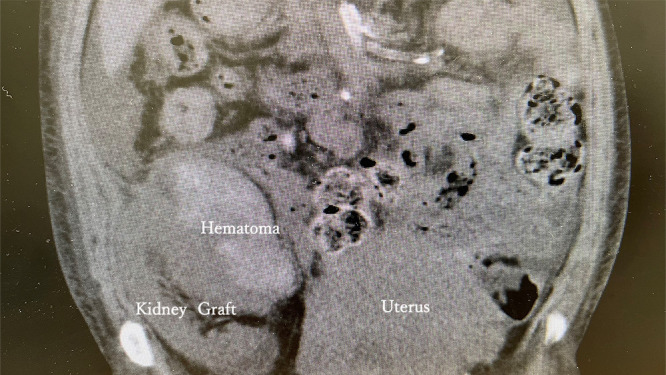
Computed tomography (vertical section) showing a hematoma around the kidney graft.
